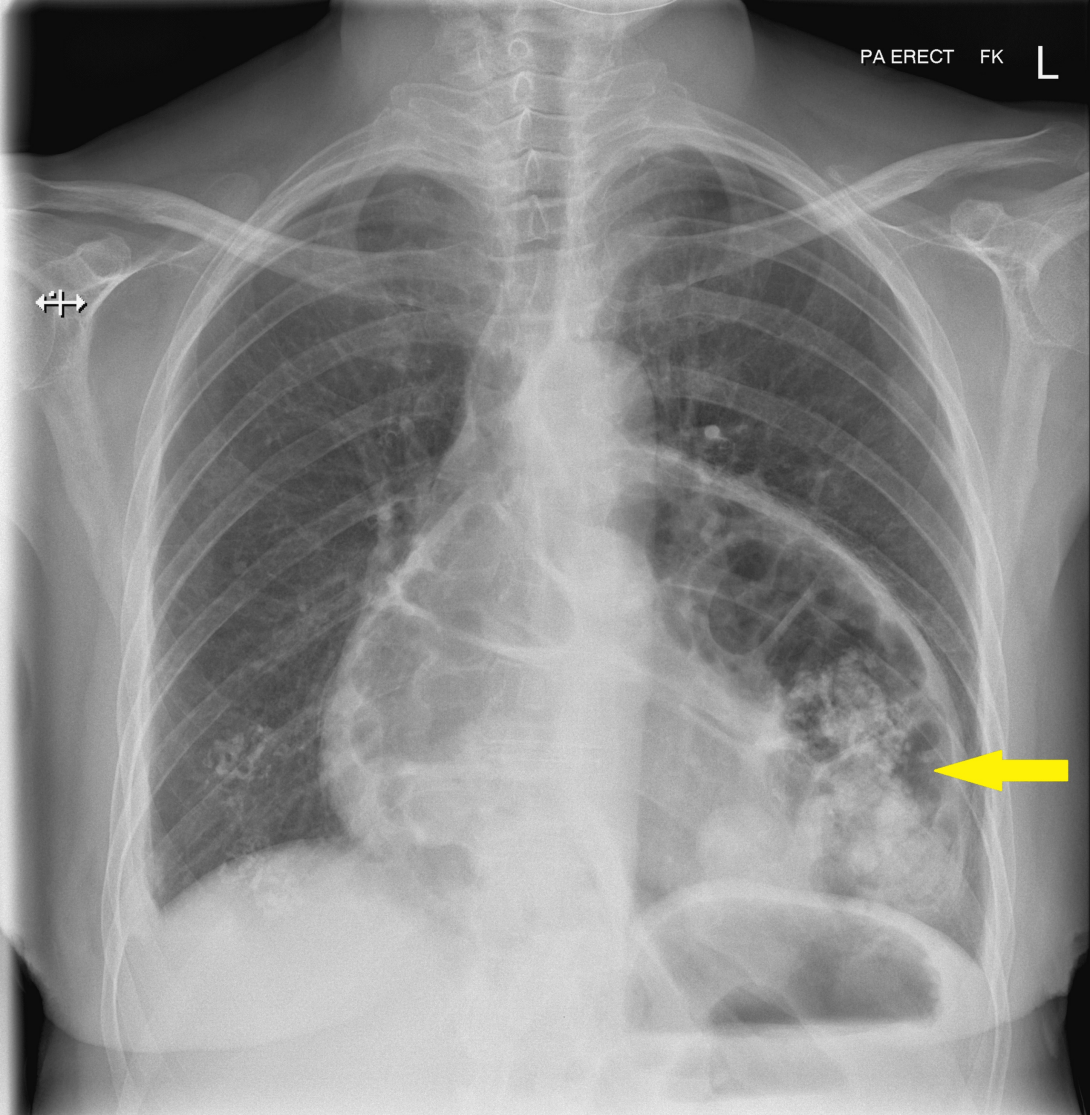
Erect chest X-ray showing large bowel loops within the mediastinum (yellow arrow) and clear lung fields
